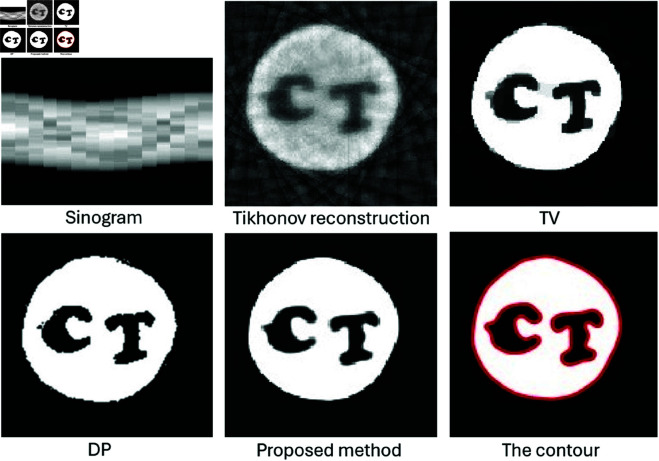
Performance comparison of the proposed method with Tikhonov, Total Variation (TV), and Dual Problem (DP) reconstructions on a real CT dataset of a carved cheese slice with sparse projections. The sinogram was acquired from 15 projections over a 360^∘^ range.
